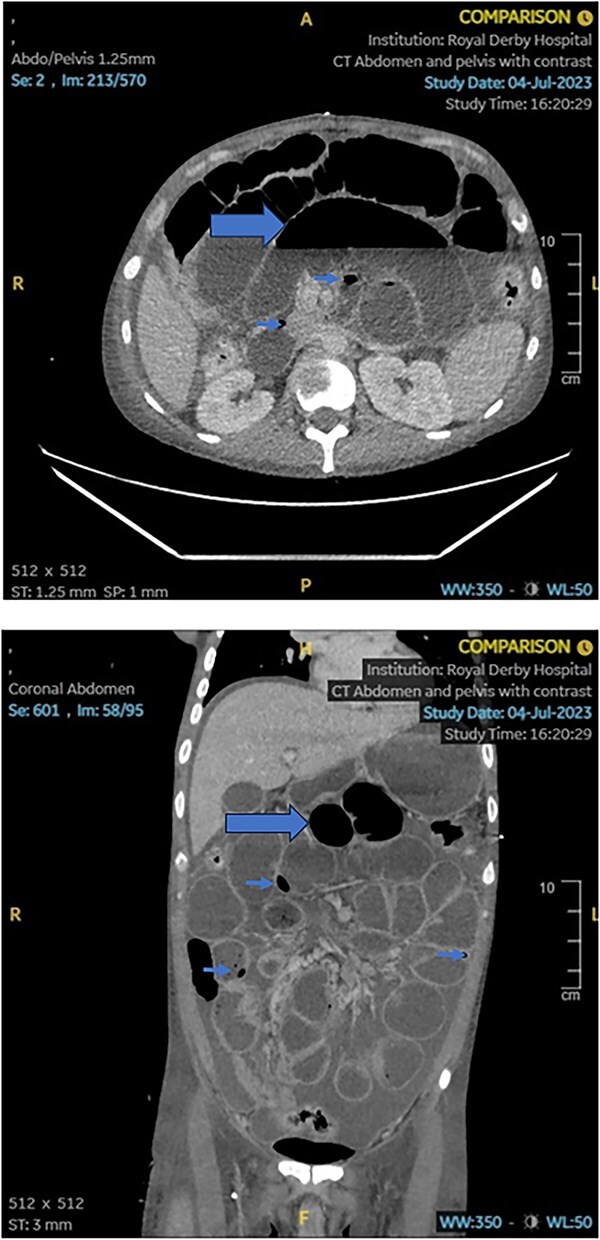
Axial and sagittal views of the patient’s initial CT scan demonstrating free intraperitoneal and intramural air (arrowheads), as well as small bowel dilatation (arrow) in keeping with an acute small bowel perforation. Free intraperitoneal fluid can also be seen.
